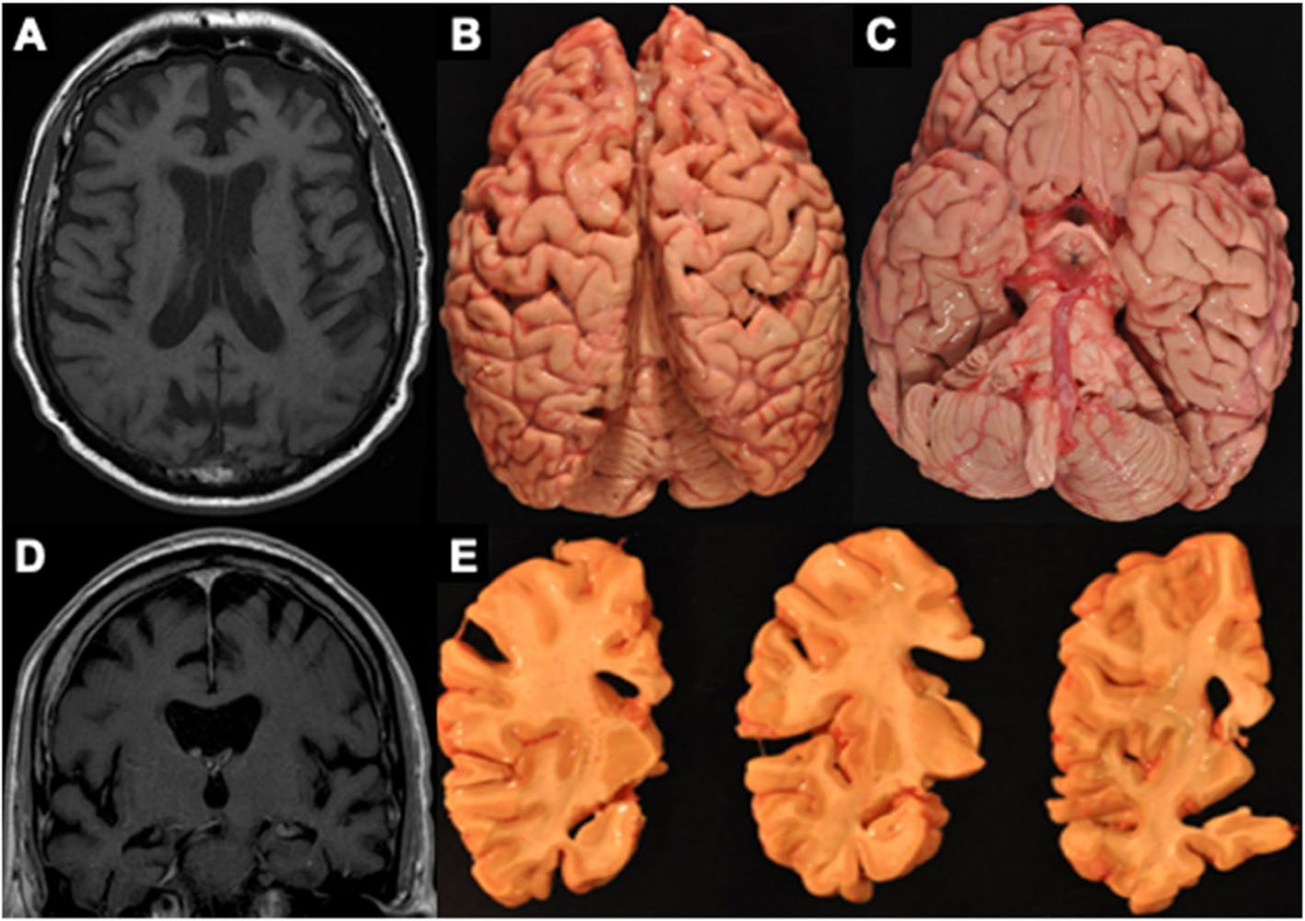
MRI and autopsy patterns of atrophy and P-tau deposition in a brain donor with CTE. The figure shows an antemortem axial (A) and coronal (D) T1 MRI sequence and corresponding gross atrophy at autopsy (B, C, E) of a male former professional American football player with CTE stage IV. The antemortem MRI scan was done when he was in his early 60’s and he died in his mid-70s and donated his brain. The antemortem MRI and neuropathological examination both showed frontal and temporal cortical atrophy (A–E) along with atrophy of medial temporal lobe structures (C, E) including the hippocampus and amygdala
